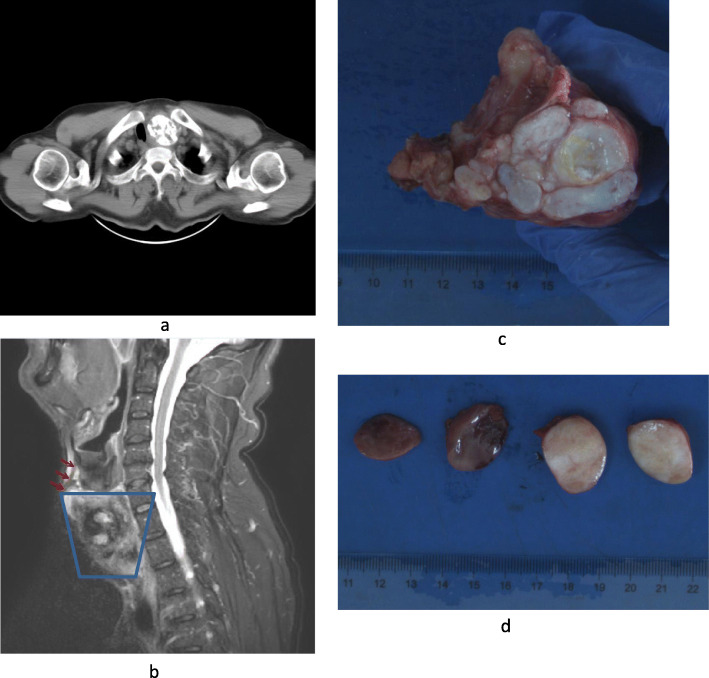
a. Computed tomography image showing a mass, 4.5 × 4.0 × 4.5 cm in size, with calcification in the left thyroid lobe and two smooth and homogeneous nodules in the right thyroid lobe. The trachea is compressed and deflected toward the right side. b. Neck MRI showed that the epicenter of the left lobe mass was in the thyroid gland and it did not originate from the surrounding thyroid cartilage (the red arrow shows the thyroid cartilage, and the blue box shows the main body of the tumor). c. Gross examination showed that a gray, lobulated mass occupied the majority of the left thyroid lobe. The cut surface appeared gray with hard consistency. d. Two homogeneous, oval nodules in the right thyroid lobe appeared gray with hard consistency
